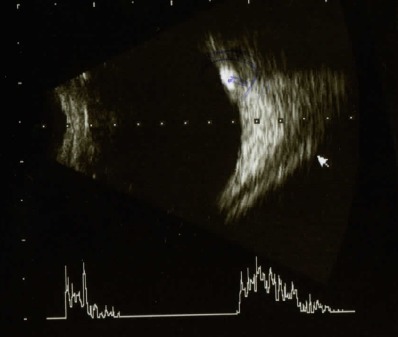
Ultrasonography right eye
